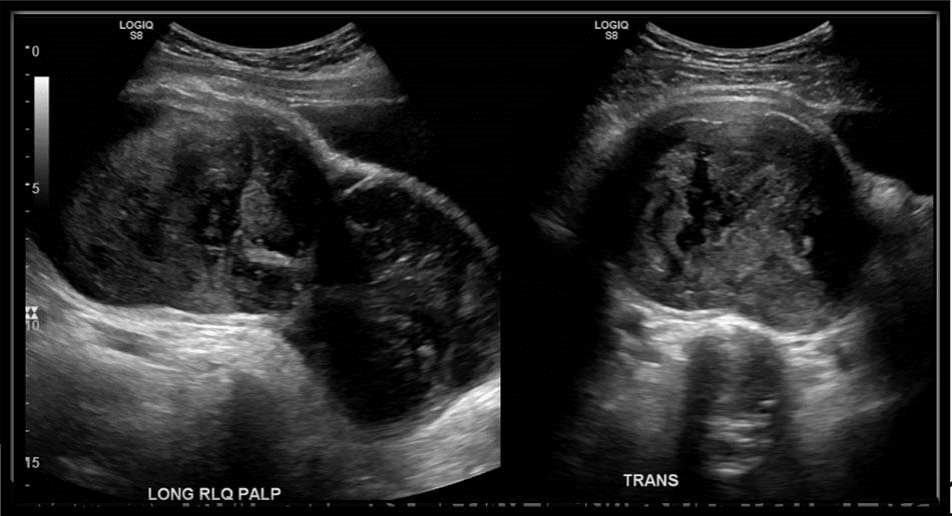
US of right lower quadrant visualizing the hypo-vascular abdominal mass longitudinally and transversely; mass measured 18.5 × 8.1 × 11 cm in the right hypogastric region with only a small amount of visible internal flow on color Doppler.
